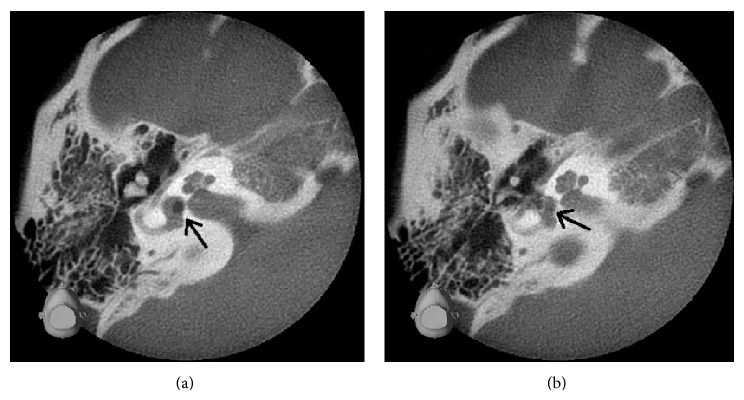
Axial views of the right ear on cone-beam computed tomography on hospital on day 1, showing a round low density area (a) and intrusion of stapes (b) (black arrows).
